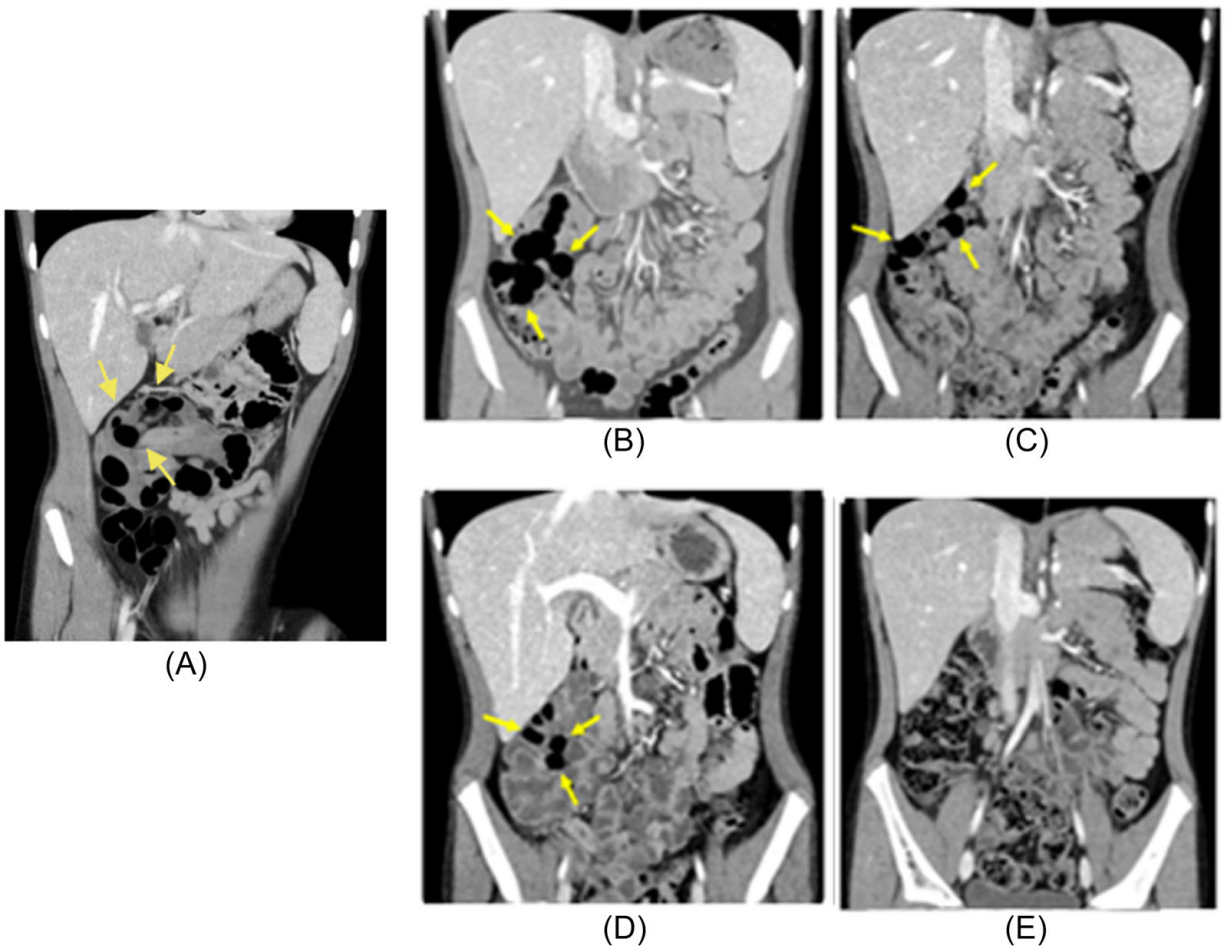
CT scans of the patient's abdomen throughout the course of treatment. Yellow arrows indicate PCC. (A) Initial CT obtained 10 months before admission. (B) Day of admission. (C) Day 10 of treatment with metronidazole and supplemental oxygen. (D) Day 28 of treatment, after 4 days of hyperbaric oxygen therapy. (E) Complete resolution of PCC 6 months after hospital admission. CT, computed tomography; PCC, pneumatosis cystoides coli.
